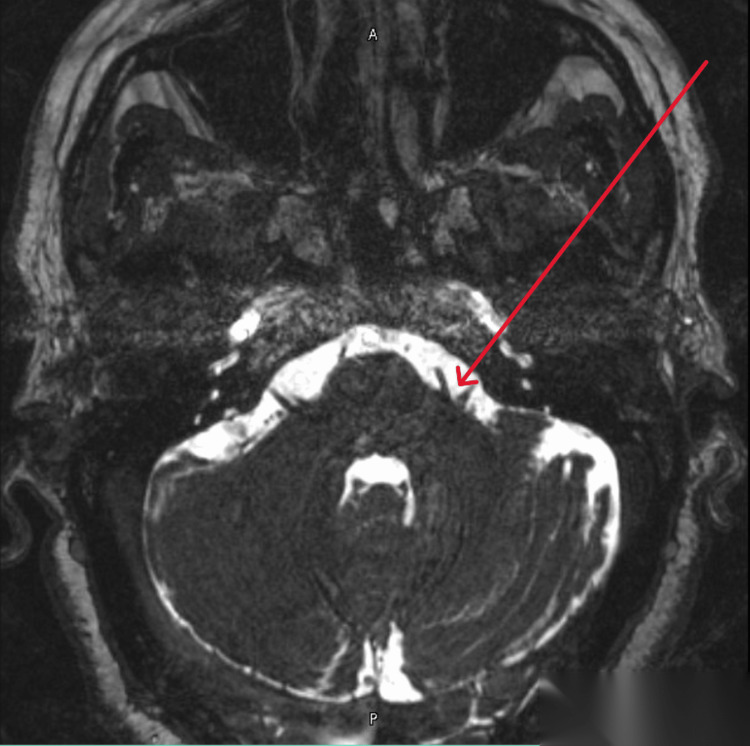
Axial high-resolution T2-weighted CISS MRI demonstrating an anomalous vascular loop of the left AICA in the left cerebellopontine angle, causing focal indentation of the cisternal segment of the left facial nerve at its root exit zone from the lower pons (arrow) AICA, anterior inferior cerebellar artery; CISS, constructive interference in steady state
